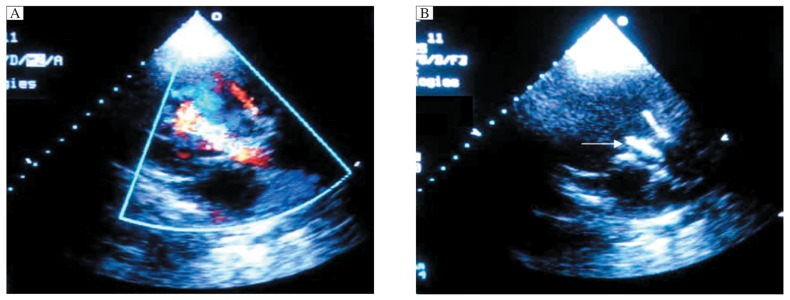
Echocardiographic image of moderate paravalvular aortic regurgitation after a 20-mm bioprothesis into an aortic root with a 19.6-mm native aortic annulus (long-axis, 2 h post procedure). A: An increased trans-aortic gradient (15.1 mmHg *vs* 3.6 mmHg pre-procedure) and moderate paravalvular aortic regurgitation were observed. B: Echocardiography showed that there was a gap between the device and the left ventricular outflow wall (arrow).
